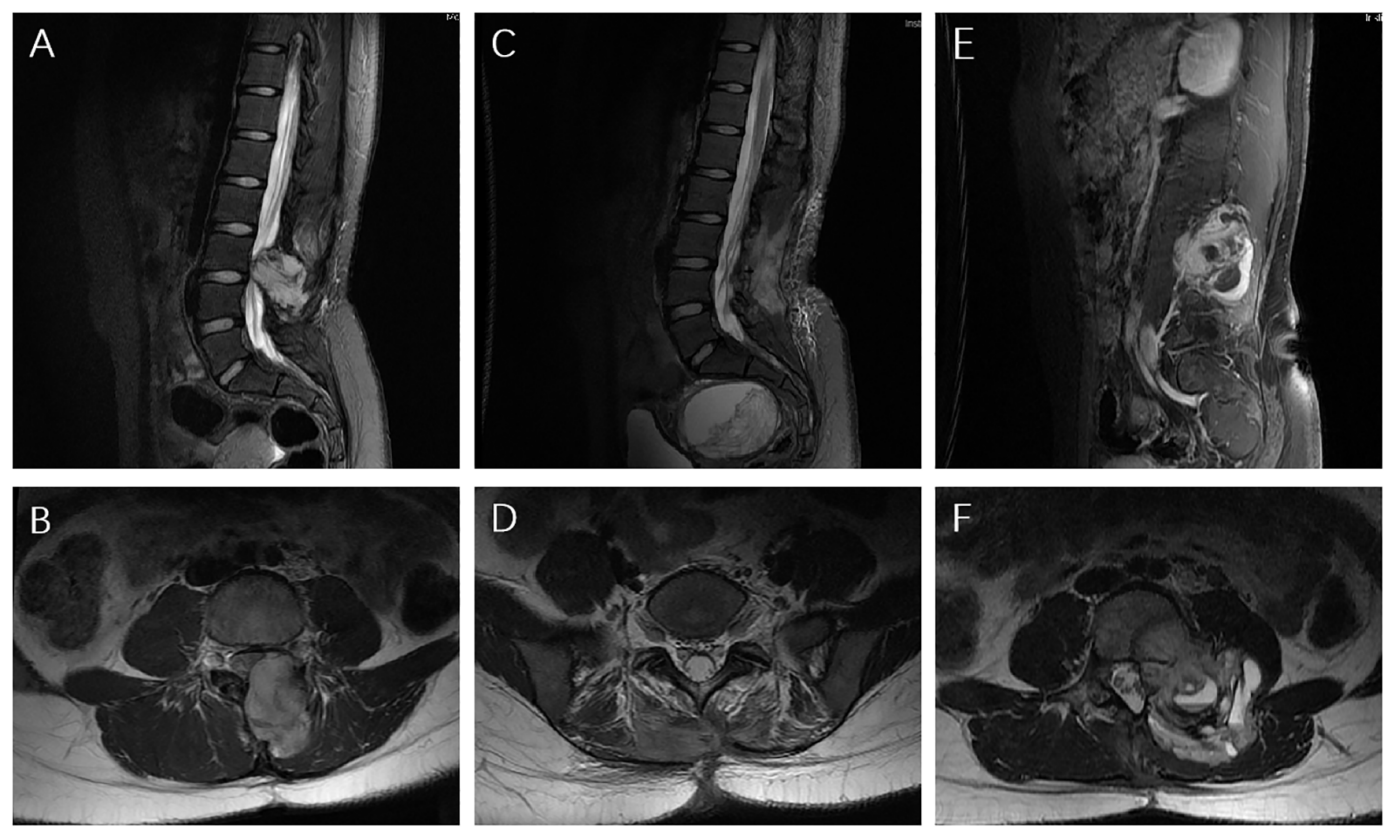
MRI images (A, B) At the first recurrence, MRI exhibited an unusual hyper-vascular mass; (C, D) Postoperative it showed no residual or occurrence of the tumor; (E, F) 8 months later, it exhibited the second recurrence.
